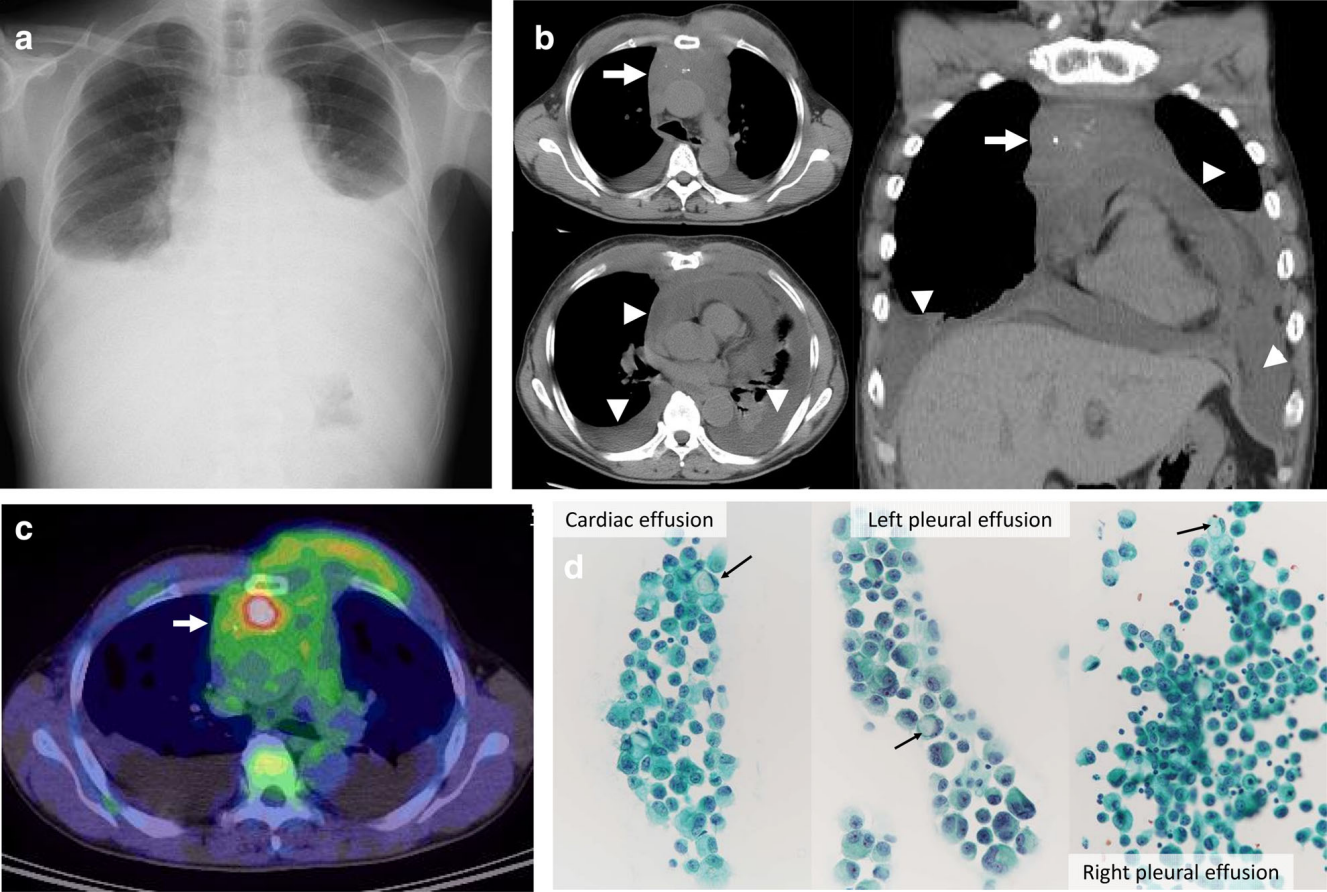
(a) Chest radiograph showing bilateral pleural effusions and cardiac enlargement. (b) Chest computed tomography (CT) scan revealed a lobulated mass measuring 87 × 83 mm in the anterior mediastinum (white arrow) with bilateral pleural effusion and cardiac effusion (white arrowhead). (c) Positron emission tomography (PET) imaging showed increased accumulation of 2‐deoxy‐2‐[18F] fluoro‐D‐glucose (^18^F‐FDG) in the anterior mediastinal mass with a maximal standardized uptake value (SUV_max_) of 7.9 (white arrow). (d) Cytological examination of bilateral pleural effusions and pericardial effusion revealed adenocarcinoma with signet ring cell‐like features (black arrows).
